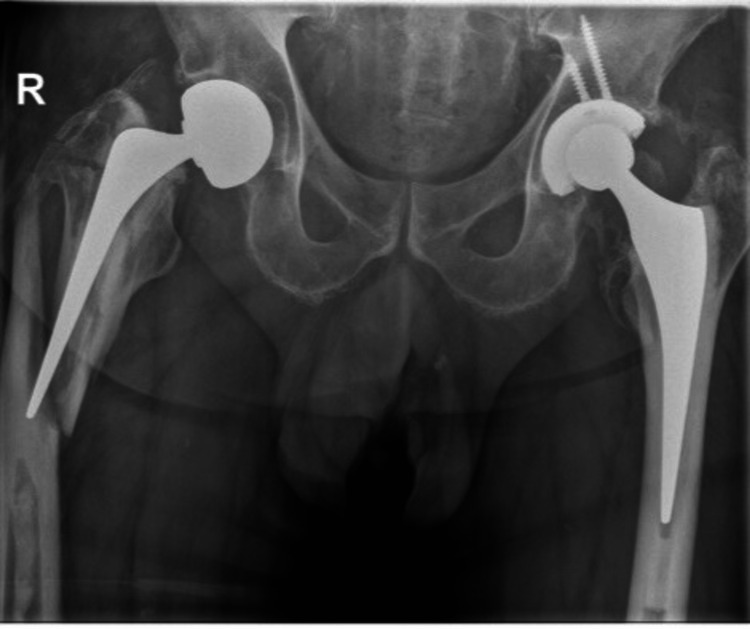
Case A, X-pelvis pre-OR X-ray showing a periprosthetic femoral fracture of the right femur, Vancouver B2 type of fracture
